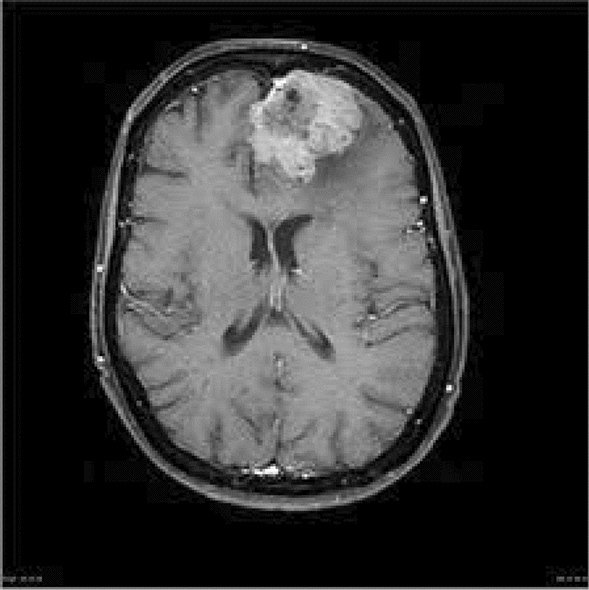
Meningioma brain MRI^1^.
